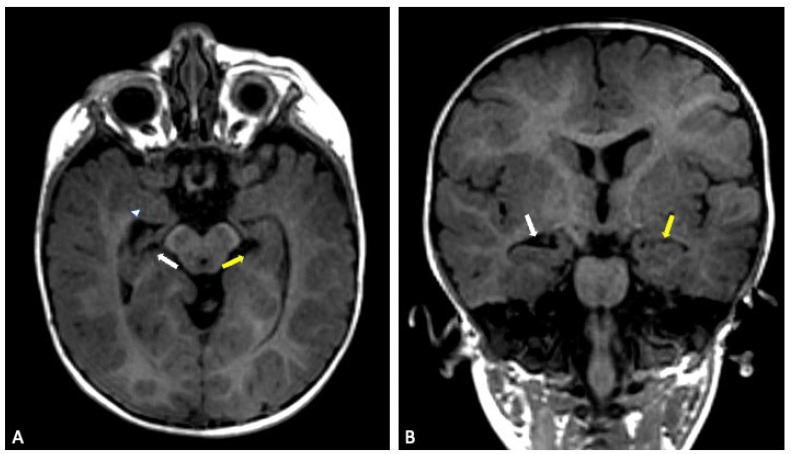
Sibling 3. Brain MRI; axial (A) and coronal (B) T1-weighted gradient echo (GE) images showing a reduced-size right hippocampus (white arrows), compared to left normal hippocampus (yellow arrows). On the right side, the compensatory enlargement of the temporal horn of the lateral ventricle can also be noted (arrowhead).
